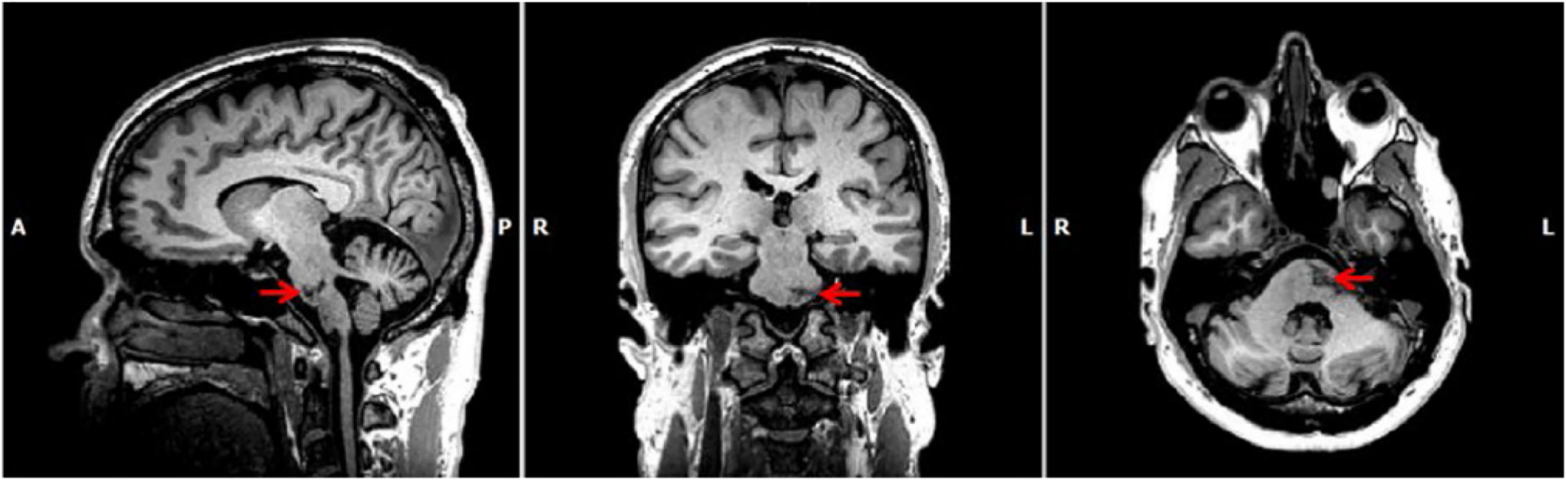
Anatomical magnetic resonance images confirming left pontine lesion (arrows). Talairach coordinates x:−7, y:−25, z:−31
